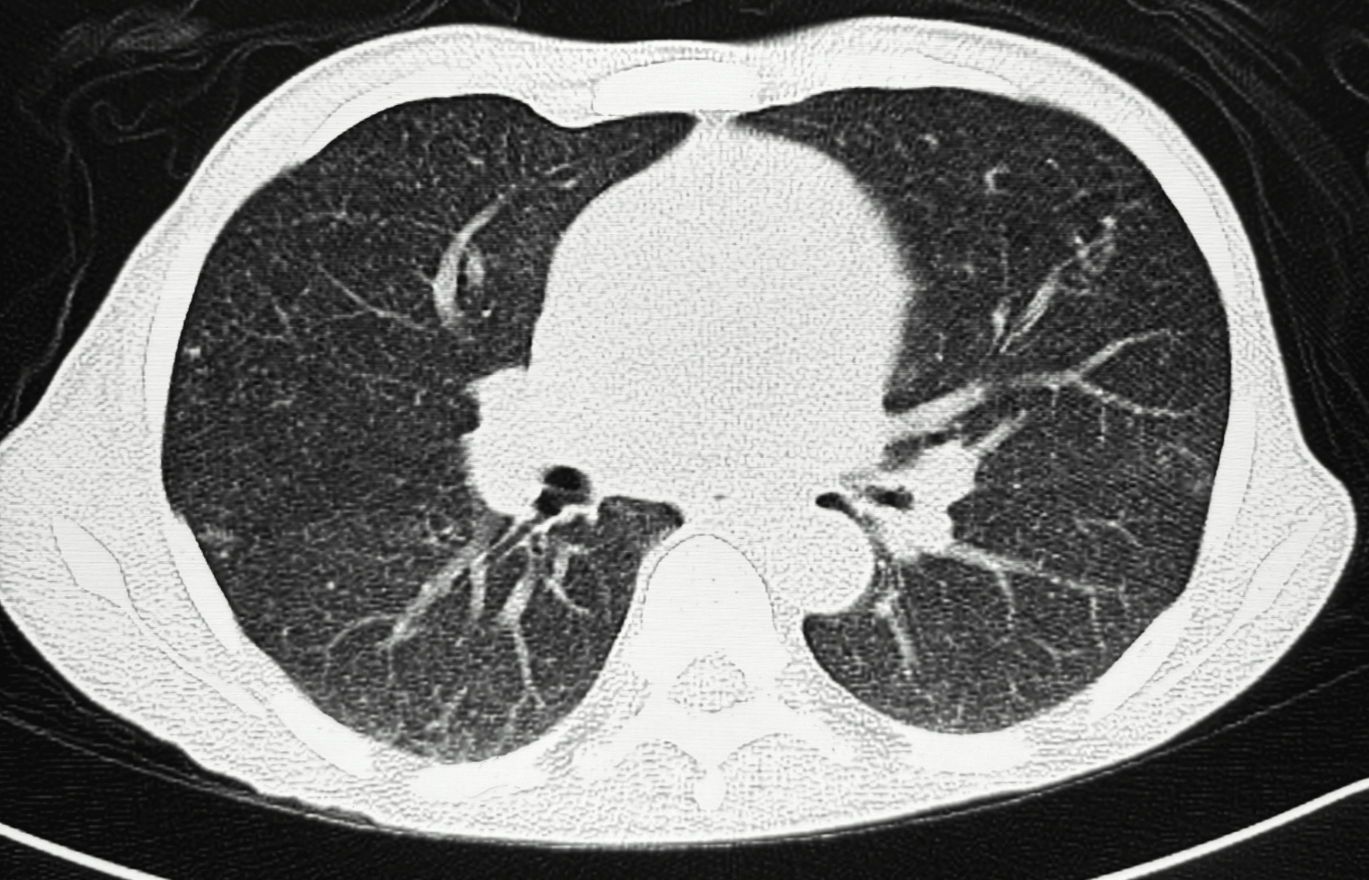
Axial CT of the chest demonstrating pulmonary nodules in a miliary pattern CT: computed tomography
